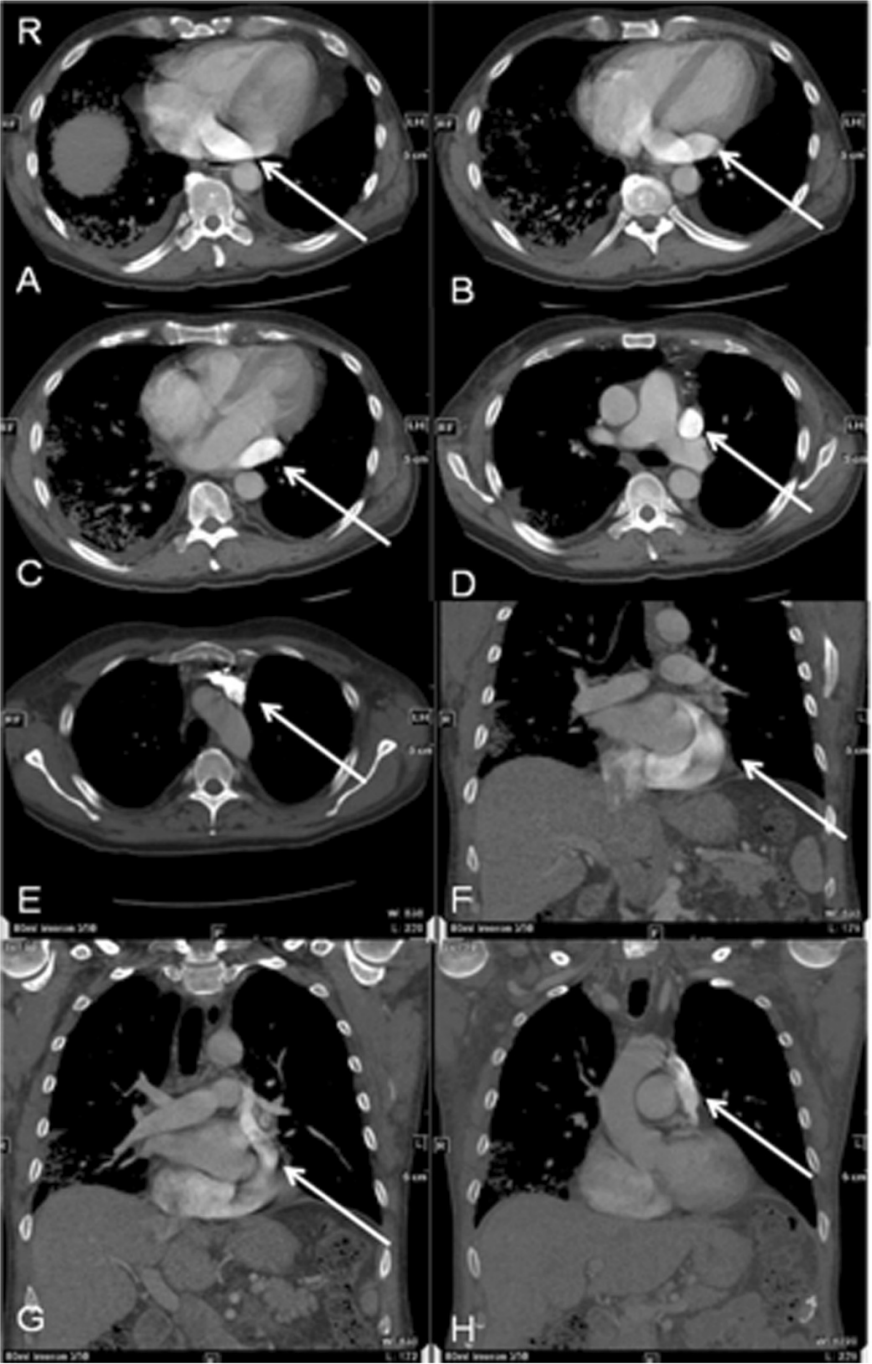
Persistent left superior vena cava in CT. A: Ostium of the persistent left superior vena cava (arrow) to the coronary sinus. B-E: Cranial-caudal rout of the persistent left superior vena cava. F-H: Coronal view of the persistent left superior vena cava.
